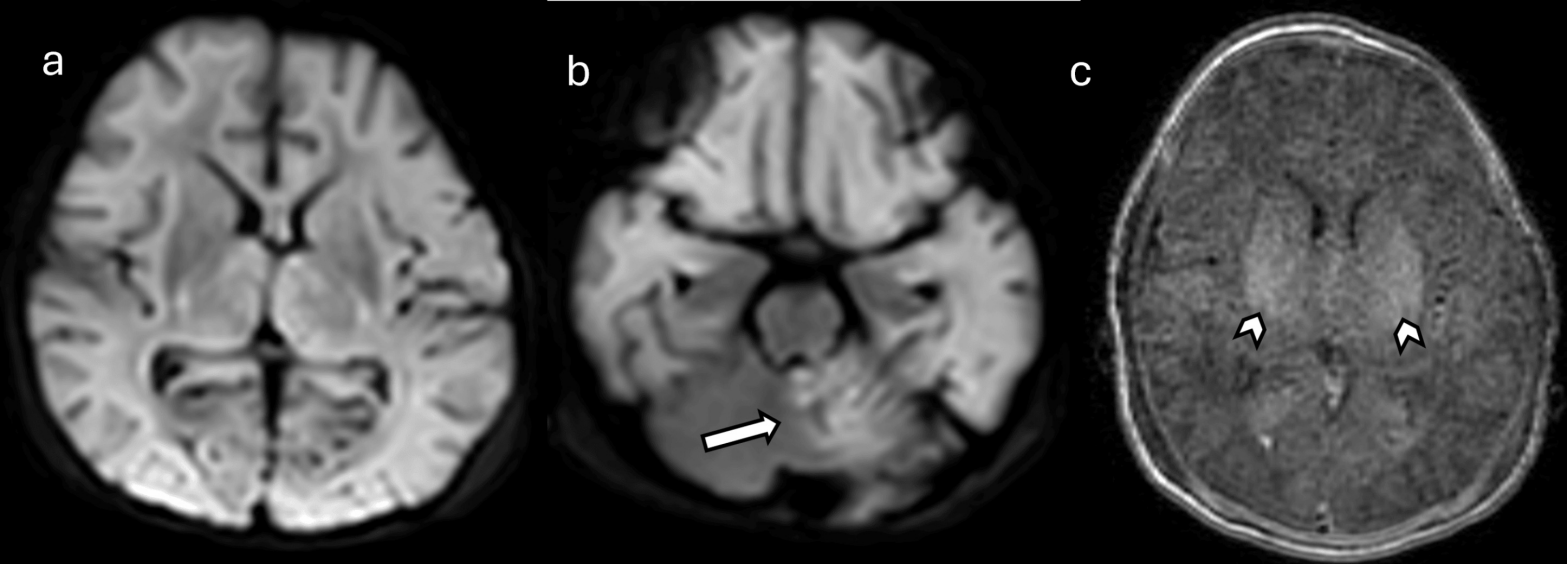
A four-day-old patient with a history of hypoxic ischemic encephalopathy, status post rewarming (a) and (b) axial DWI and (c) axial T1W images showing global injury pattern with widespread, extensive restricted diffusion involving the gray and white matter of both cerebral hemispheres, corpus callosum thalami, the corticospinal tracts, basal ganglia, and the left cerebellar hemisphere (arrow). Note the internal capsule posterior limb signal loss on T1W (arrowheads). Images obtained by the authors from the institutional PACS. All images are de-identified and used in accordance with institutional policy. DWI: diffusion-weighted imaging; T1W: T1-weighted; PACS: picture archiving and communication system
